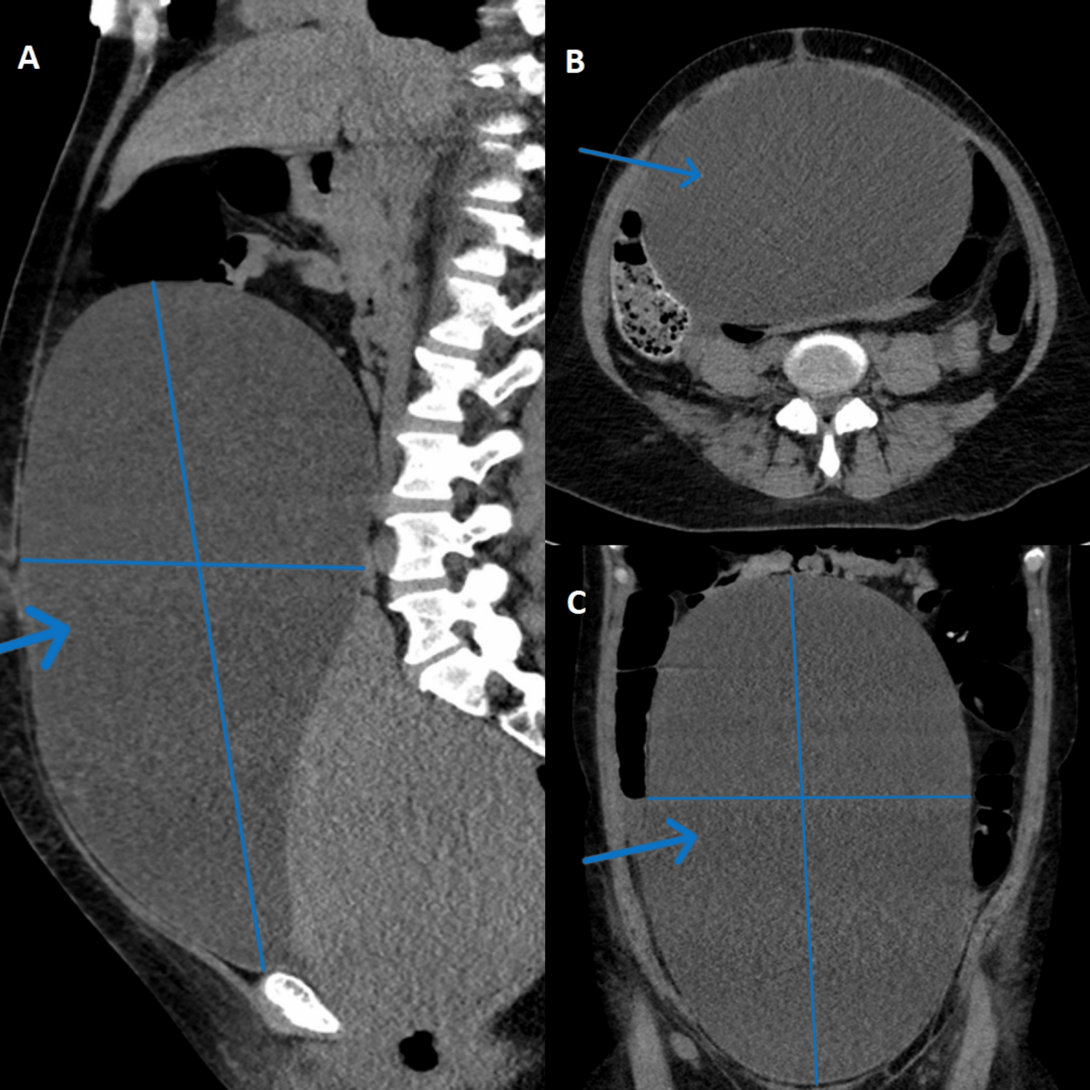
Blue arrows in pictures A, B, and C point to an enlarged urinary bladder in sagittal, cross-sectional, and coronal views, respectively, in the non-contrast computed tomography (CT) scan of the abdomen and pelvis.
